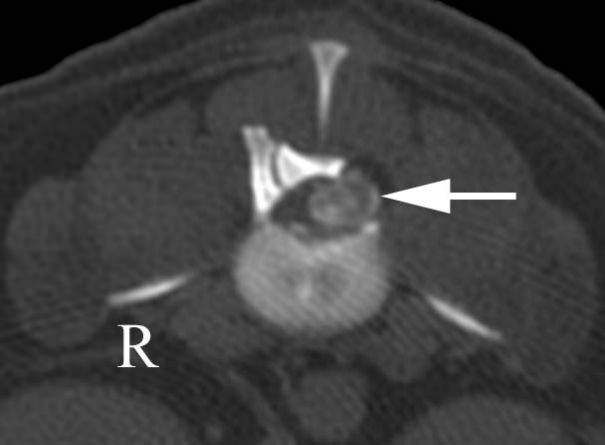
CT scan in bone window through L2-3 three weeks after left sided hemilaminectomy. The scan reveals calcified material (arrow) in the spinal canal compressing the spinal cord laterally towards the right. The calcified material was presumed to represent a recurrent disc extrusion that was confirmed at surgery.
